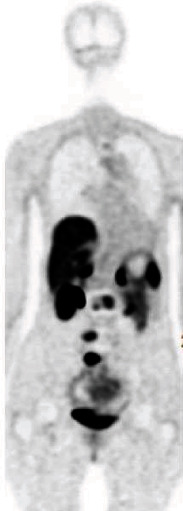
PET/CT GA-68 dotatate scan showing a modest response to therapy with no new lesions identified.
